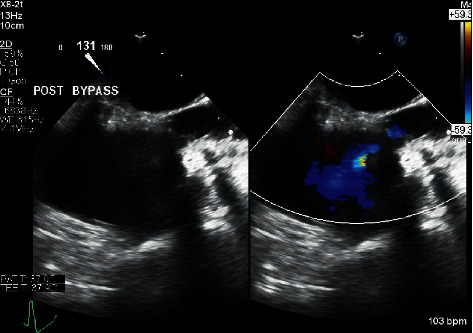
The image in the left panel shows the two-dimensional midesophageal bicaval TEE view after surgical revision. The image in the right panel is the same with the addition of color flow Doppler demonstrating improved flow from the SVC into the right atrium.
